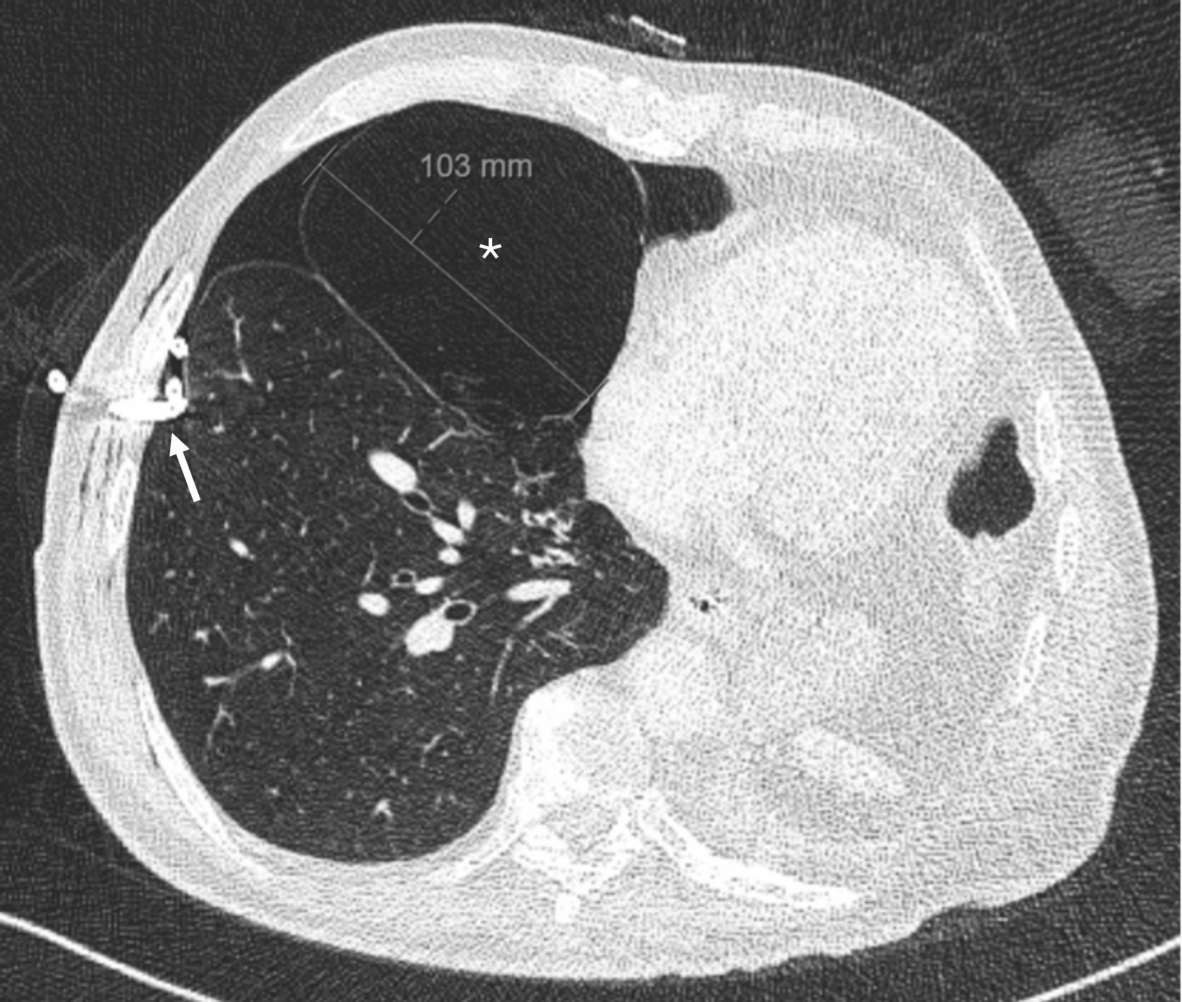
Chest CT scan. Axial chest CT scan demonstrating a large emphysematous bulla in the right middle lobe (asterisk), measuring approximately 103 mm in its greatest diameter. The arrow indicates the pigtail chest tube in situ.
